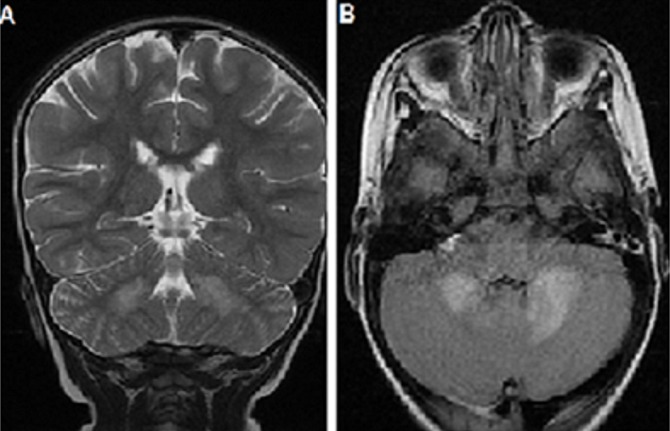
(A): IRM cérébrale séquence T2 axiale; (B): T2 FLAIR axiale montrant des hyper-signaux cérébelleux et de la substance blanche sus-tensoriel
